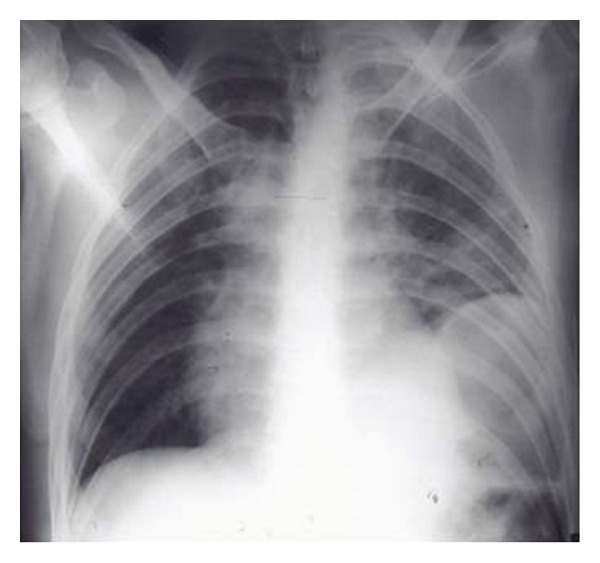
A CXR on a patient with thoracic trauma following a road traffic accident. Diagnosis of a hemopneumothorax was made, but before proceeding to a chest drain placement, a CT scan was obtained as a part of a work up for multiple traumas, which showed a diaphragmatic rupture and herniation of stomach into the left hemithorax explaining the air/fluid at the left lung base.
